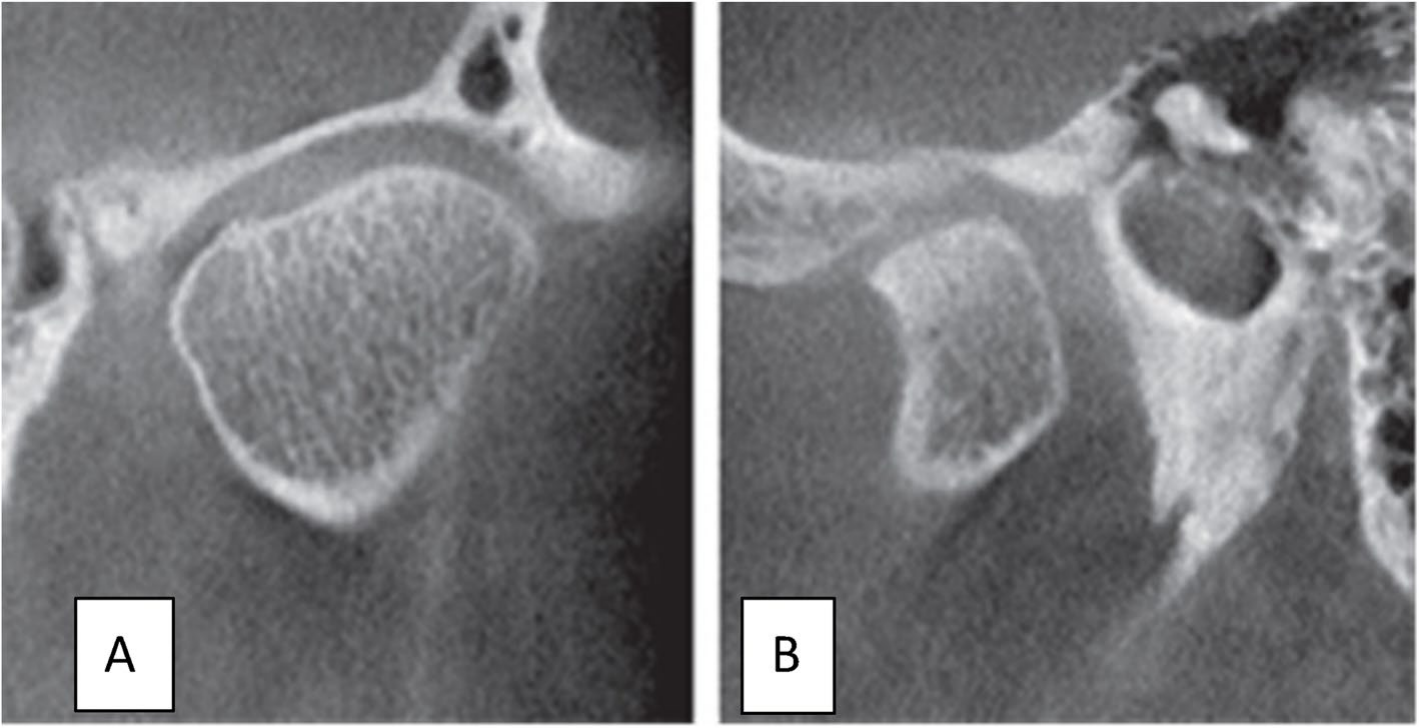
Coronal (A) and Sagittal (B) CBCT view showing Sclerosis
